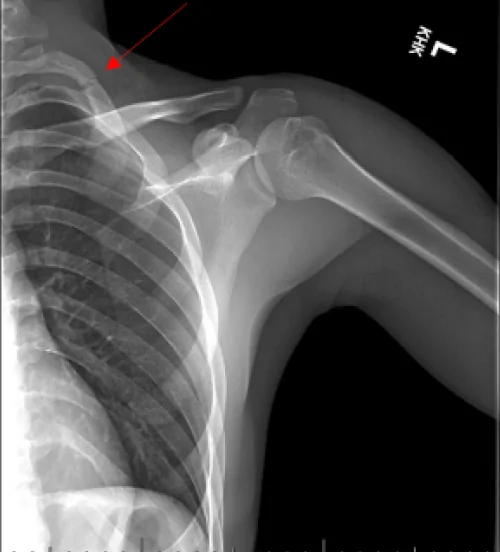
Scapula radiograph demonstrating incidental finding of first rib fracture marked by arrow, in the case of a teenage male injured while weightlifting.
